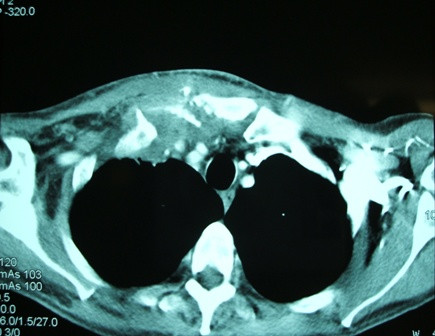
The final computed tomography scan established the correct diagnosis, showing erosive changes within the joint.
